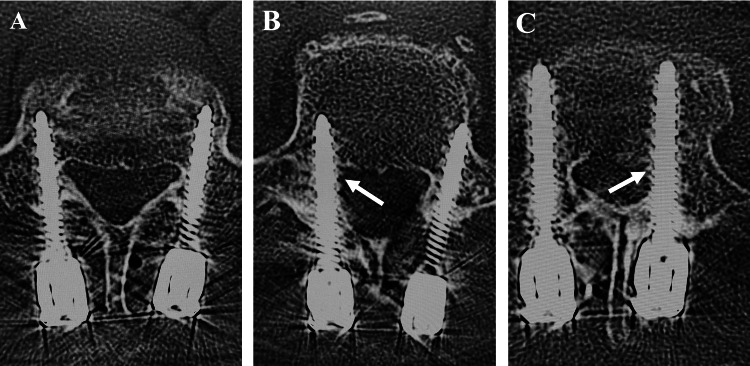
CT evaluation The deviation of the CBT screw was evaluated on CT one week after surgery using the Gertzbein-Robbins grade system [17]. A: Grade A: no breach of the cortical layer of the pedicle. B: Grade B: breaches less than 2 mm. C: Grade C: breaches of 2 mm or more but less than 4 mm. CT: Computed tomography; CBT: cortical bone trajectory
